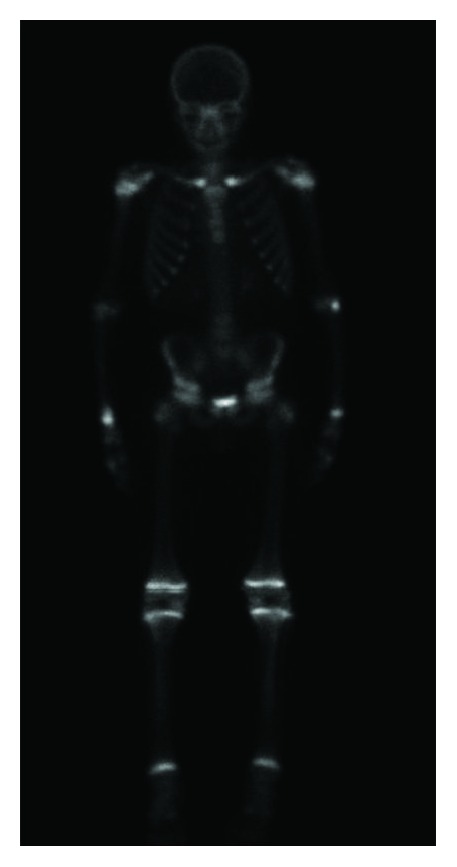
Bone gallium/scintigraphy demonstrated hypercapation at the clavicles bilaterally as well as hypercaptation at the lateral side of the right trochanter.
